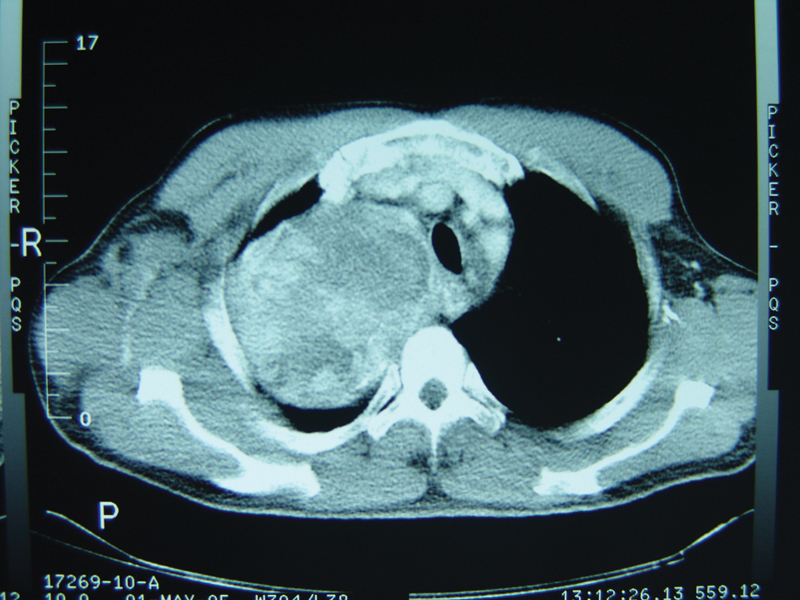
Computed tomography of giant tumor.
